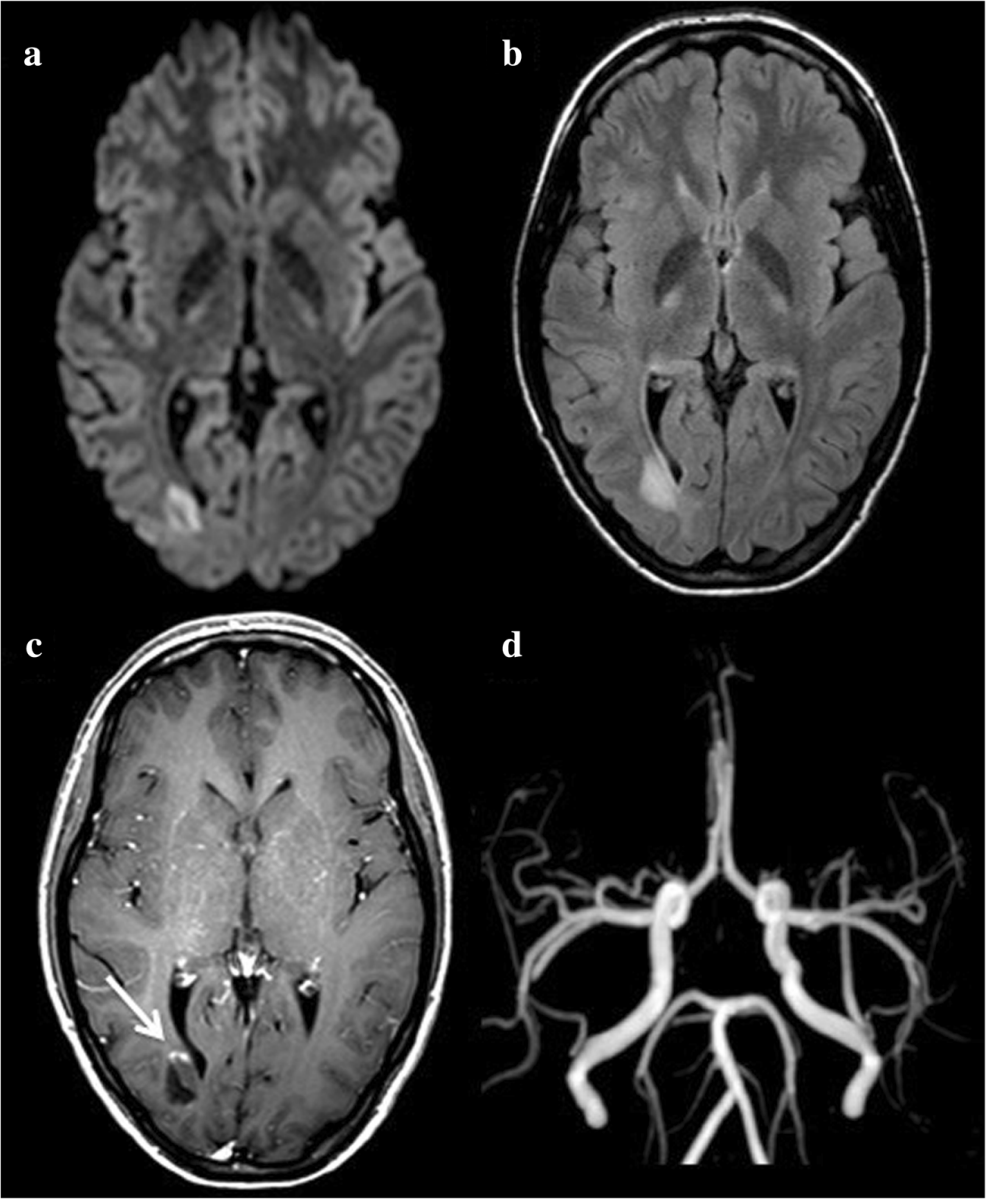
A 17-year-old woman with multiple sclerosis presenting with left homonymous hemianopsia. DWI shows ring hyperintensity (a). The lesion appears as hyperintensity on FLAIR (b) with gadolinium enhancing (c, arrow). No arterial occlusion (d)
